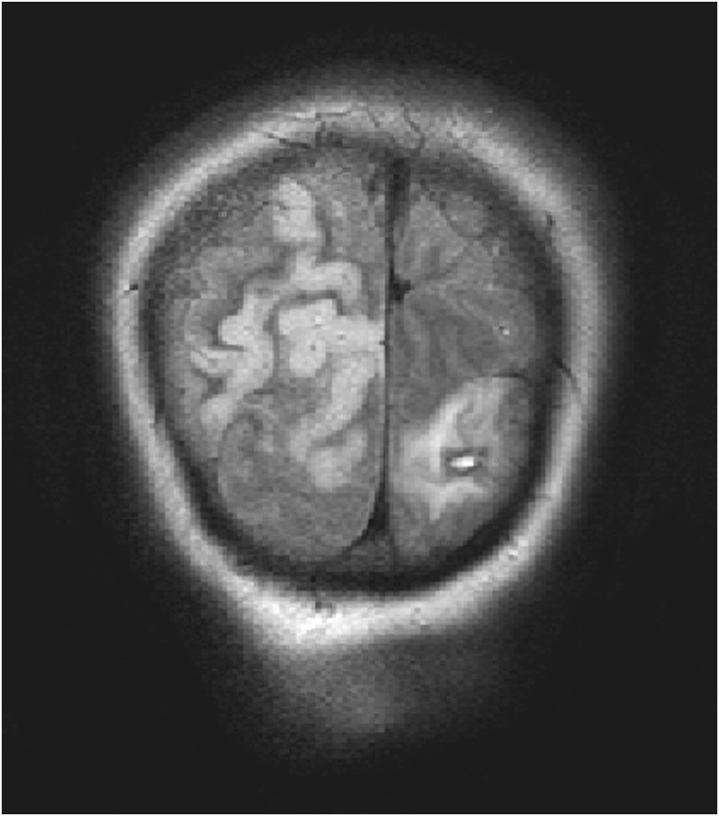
Primary coronal T2-weighted FLAIR image.
